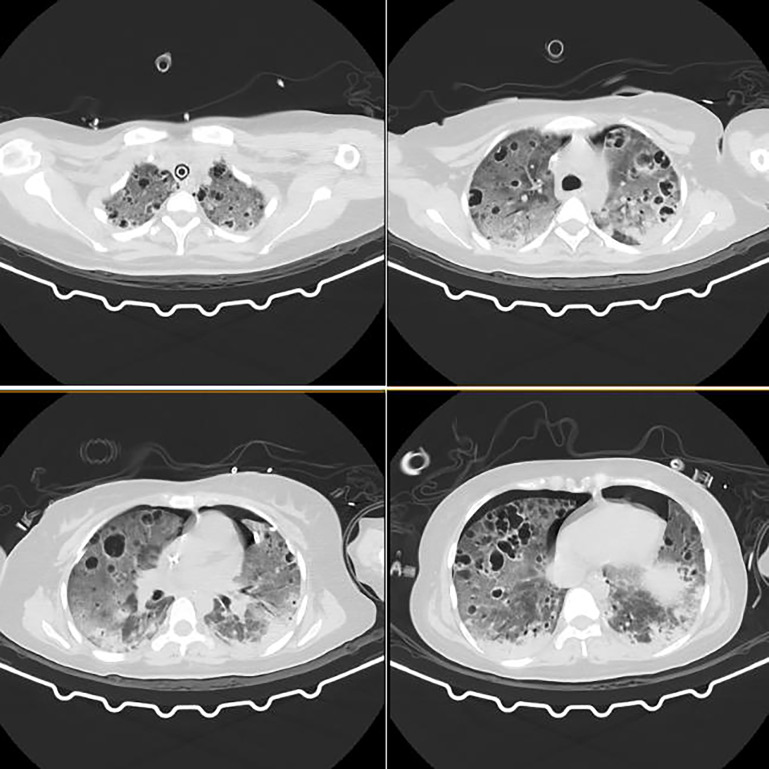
Chest computed tomography imaging on day 7 in the SICU. A chest CT scan demonstrated diffuse pulmonary cystic lesions with ground-glass opacities, multiple solid nodules bilaterally, and pleural effusion with consolidation and atelectasis in both lower lobes.
